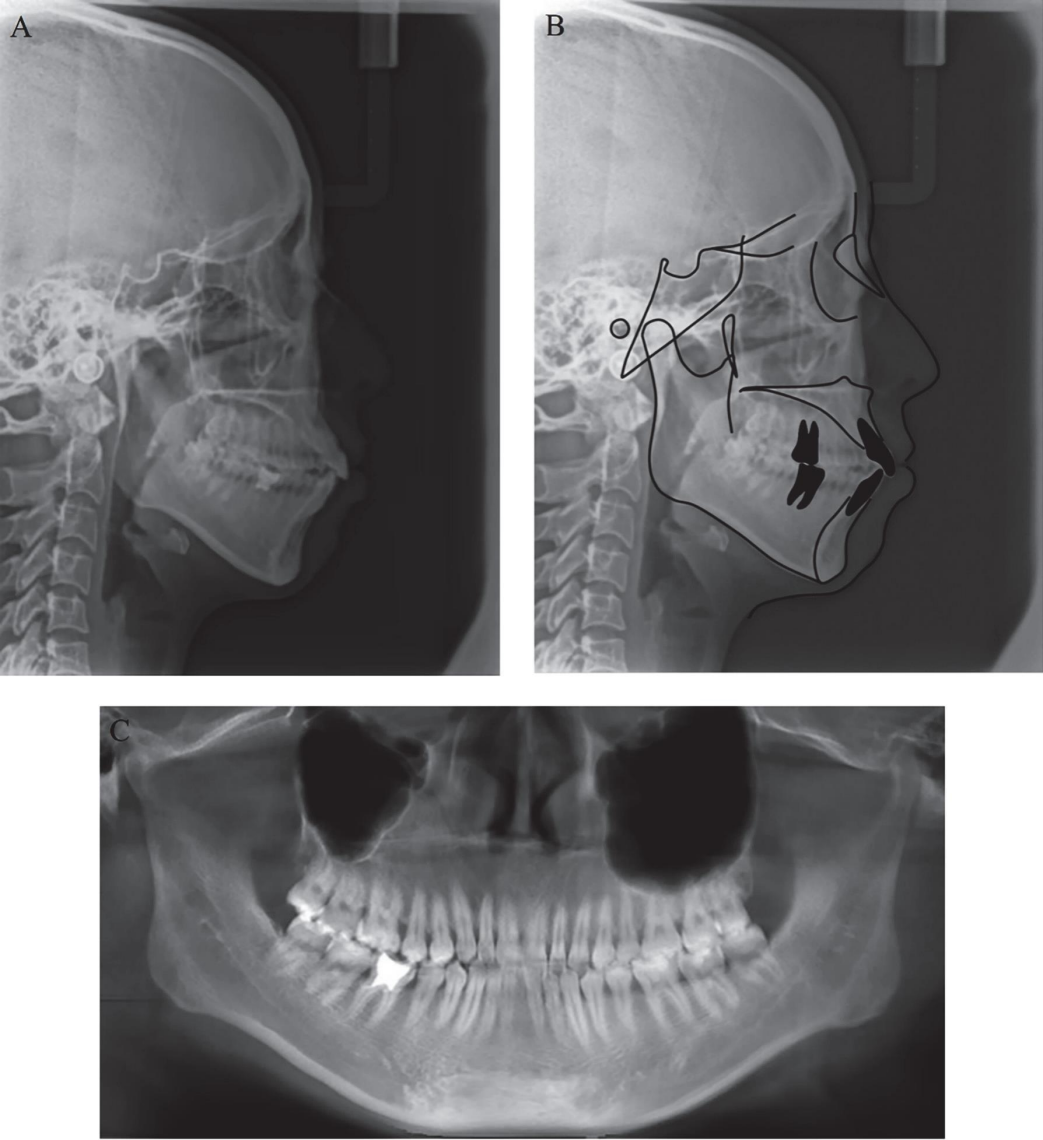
(A) Pretreatment cephalometric radiograph; (B) cephalometric tracing; and (C) panoramic radiograph.
